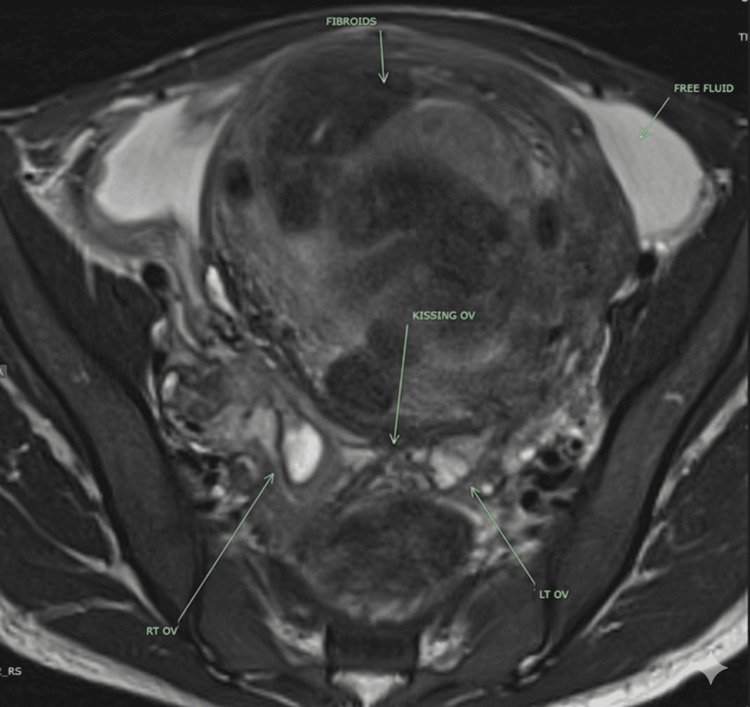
MRI showing kissing ovaries and posterior endometriotic plaque (annotated) in Case 1 after the treatment; ascites has resolved
